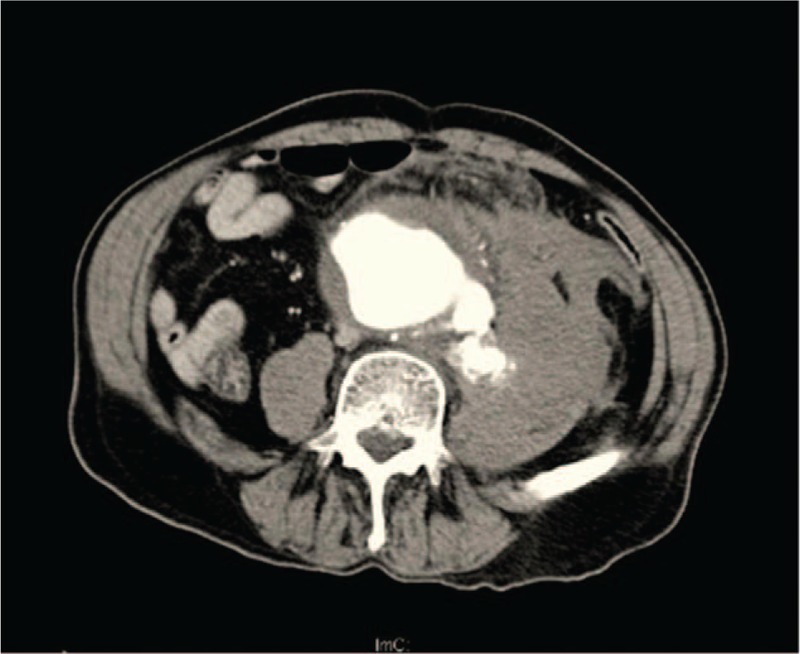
CT scan shows rAAA with huge hematoma in the left side of retroperitoneal space. CT = computed tomography, rAAA = ruptured abdominal aortic aneurysm.
